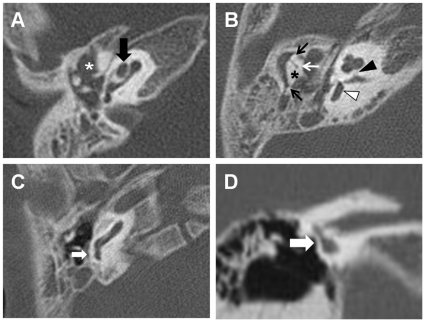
Computed tomography findings of inner ear anomalies typically seen in patients with CHARGE syndrome. (A) Cochlear hypoplasia is shown by a black arrow (Patient 1). (B) Bony cochlear nerve canal is obliterated (black arrowhead) and complete aplasia of the semicircular canals is seen (white arrowhead). The incus is dysmorphic and slightly rotated state (asterisk). Ankylosis between the incus and malleus (white arrow) and between the ossicles and epitympanic bone (black arrows) is shown (Patient 4). (C) Bony obliteration of the round window is seen (small white arrow) (Patient 6). (D) Oval window atresia is seen on the coronal image (large white arrow) (Patient 2).
